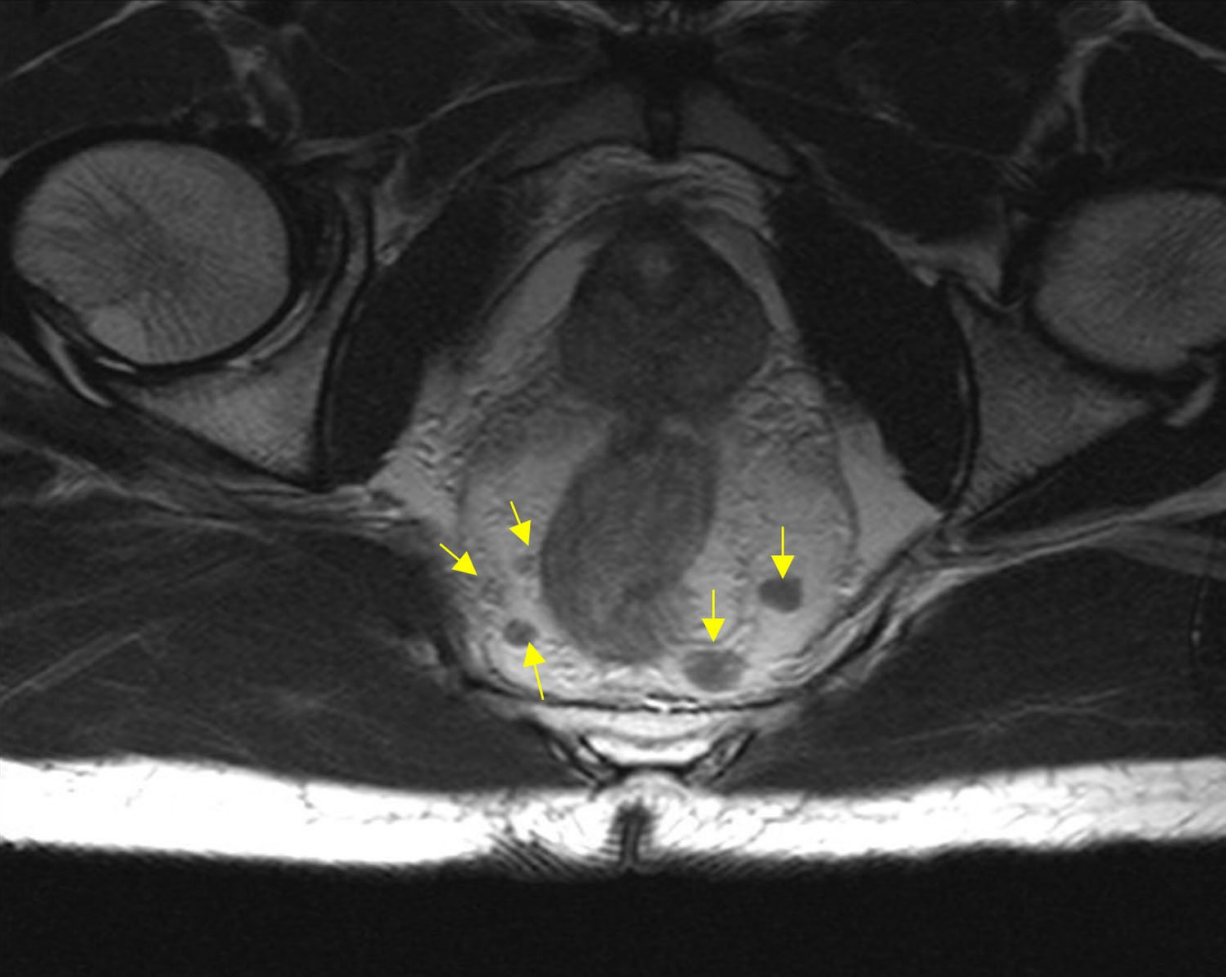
Staging MRI scan which shows multiple lymph node metastases as denoted by arrows
